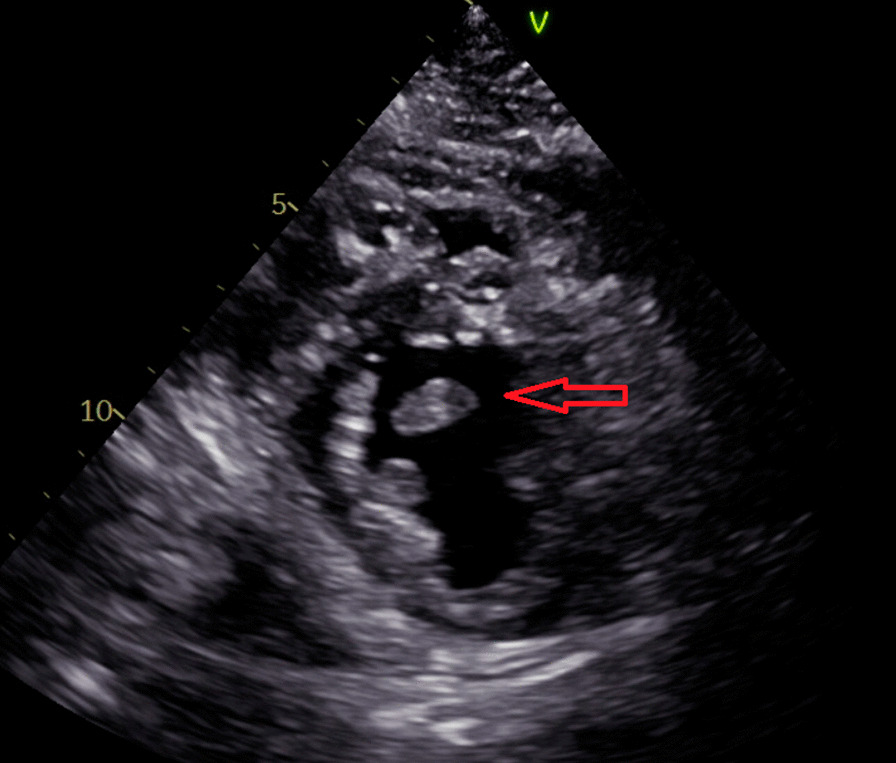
Echocardiography in parasternal short axis view performed post-operatively showing a large thrombus in the left ventricle
